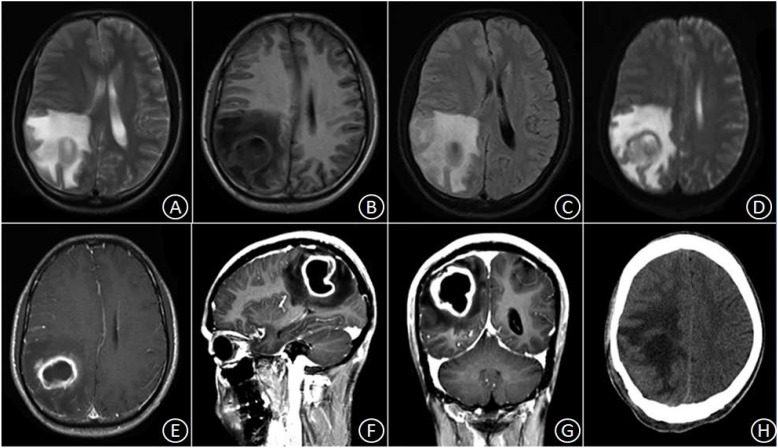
In the right parietal lobe, an irregularly circular space-occupying lesion was found with slightly long T1 and slightly long T2 signal shadows, in which sheet-like, long T1 and T2 signals were observed surrounded by large patches of oedema (A–C). DWI signals were apparently enhanced, and the marginal ring wall appeared to have a relatively even thickness, which manifested as slightly short T1 and T2 signals (D). After enhancement, the ring wall exhibited apparent signal intensification (E–G). Three weeks after the surgery, the space-occupying lesion disappeared, but patches of oedema with blurred boundary remained (H).
